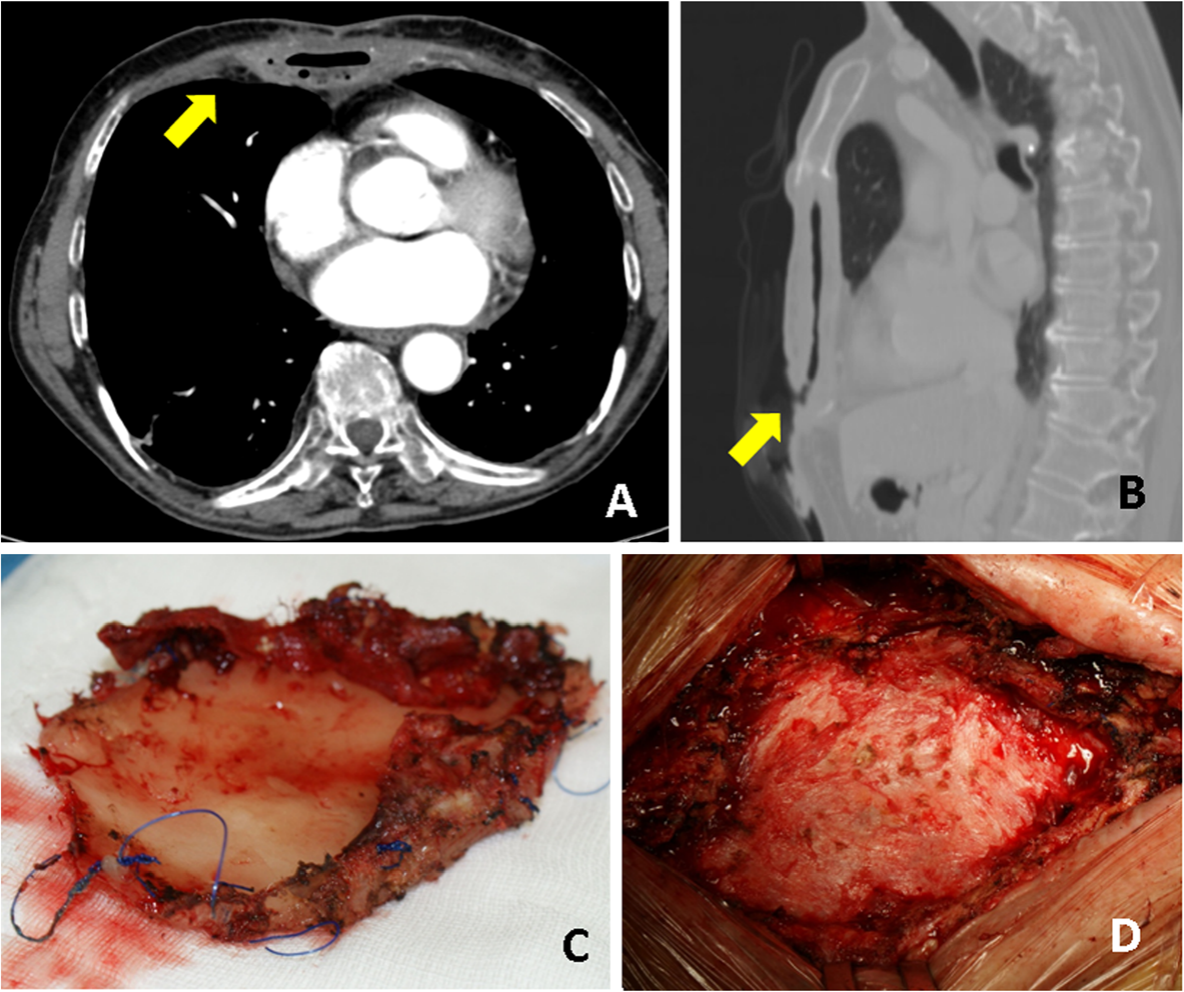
A-B) Computed tomography scan shows the infected prosthesis surrounded by multiple leveled hyperdense fluid (arrows) C) Methyl-methacrylate plate after removal; D) Intraoperative finding after prosthesis removal.
